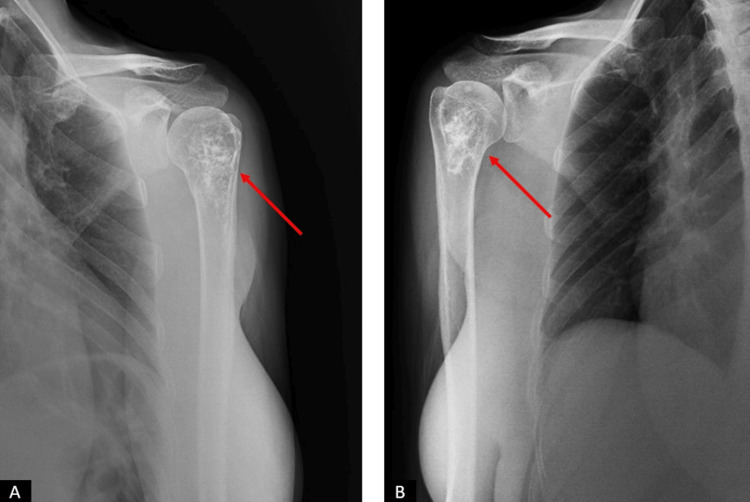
The patient's initial radiographs. (A) Left shoulder AP view. (B) Right shoulder AP view. The lesions in the humeral heads are indicated with red arrows.
